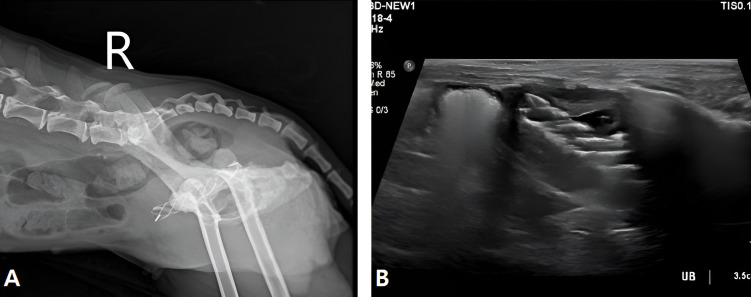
Post-procedural findings following urethral stent placement. (A) Lateral radiographic image showing appropriate positioning of the urethral stent. (B) Ultrasonographic image demonstrating the stent within the urethral lumen with maintained patency.
